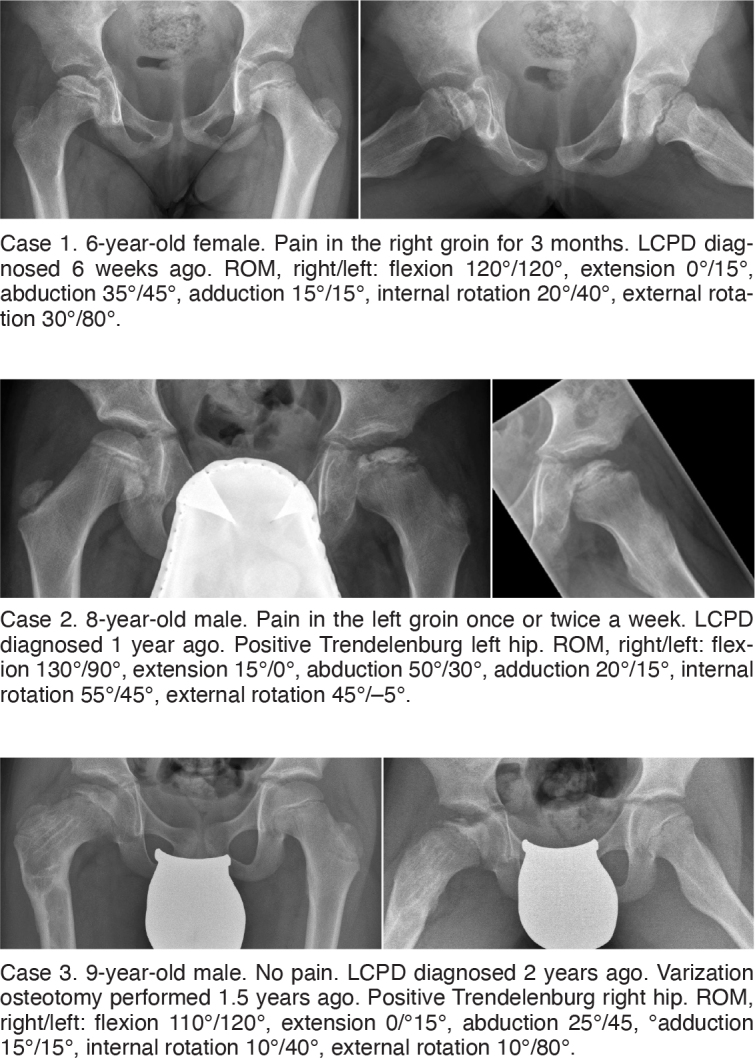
Patient cases covering the initial stage (case 1), fragmentation stage (case 2) and re-ossification stage (case 3) of Perthes disease. Frontal and frog leg/lateral radiograph and clinical characteristics. ROM = range of motion.
